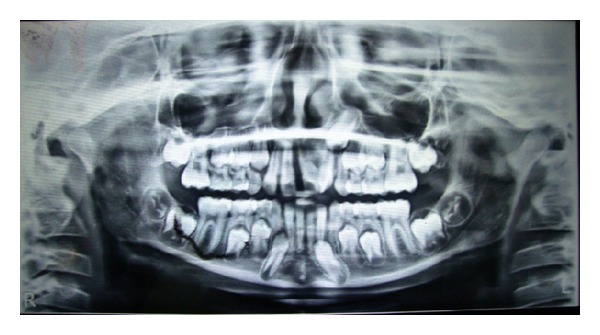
Pretreatment panoramic radiograph showing incomplete fusion of 21 and 22 at crown level.
